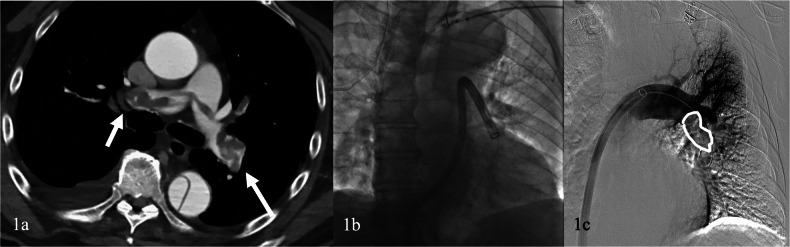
A 68-year-old patient presented to the emergency department hypotensive with saturation drop. a Computed tomography angiography confirmed the presence of bilateral extensive pulmonary emboli (arrows). b Treatment with INARI 20 F device followed with numerous bilateral aspirations. c Contrast injection confirmed the reduction of clot burden (white draw line). The nitinol expanding system was not required
